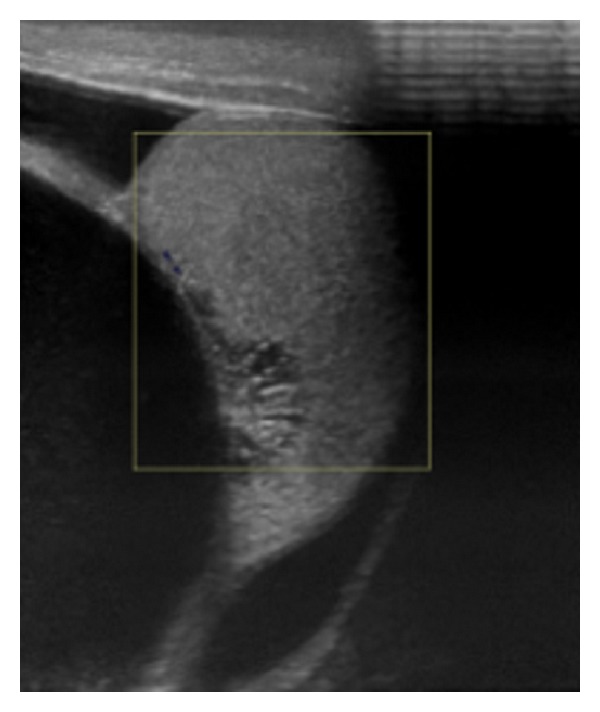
Ultrasound image of the right testicle cystic mass.
